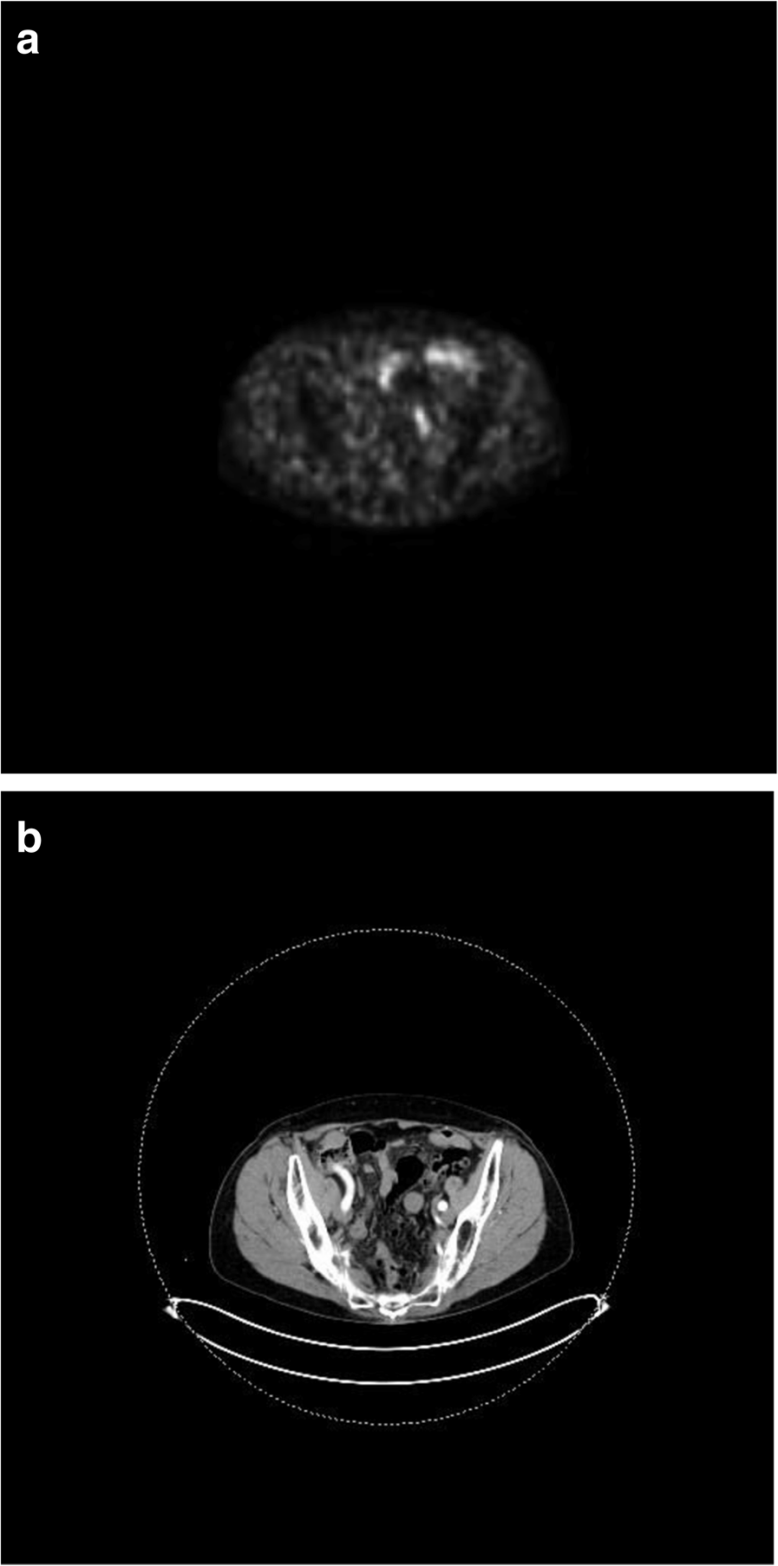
a Absence of metabolic activity on Octreo-PET/CT (axial). b Corresponding image on CT scan
